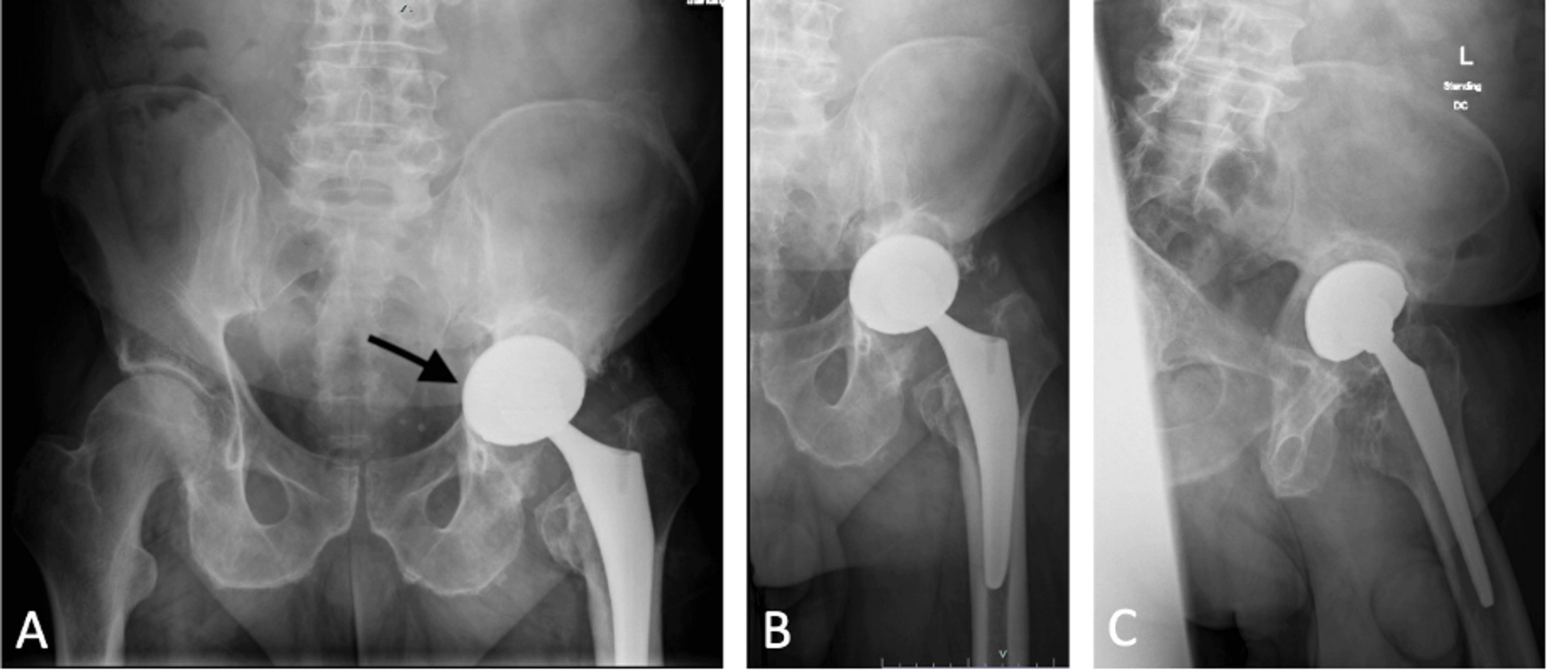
Pelvis X-rays (A) AP pelvis; (B) AP left hip; (C) lateral left hip radiographs reveal a press-fit THA with acetabular protrusio (black arrow) and medial wall loss.
